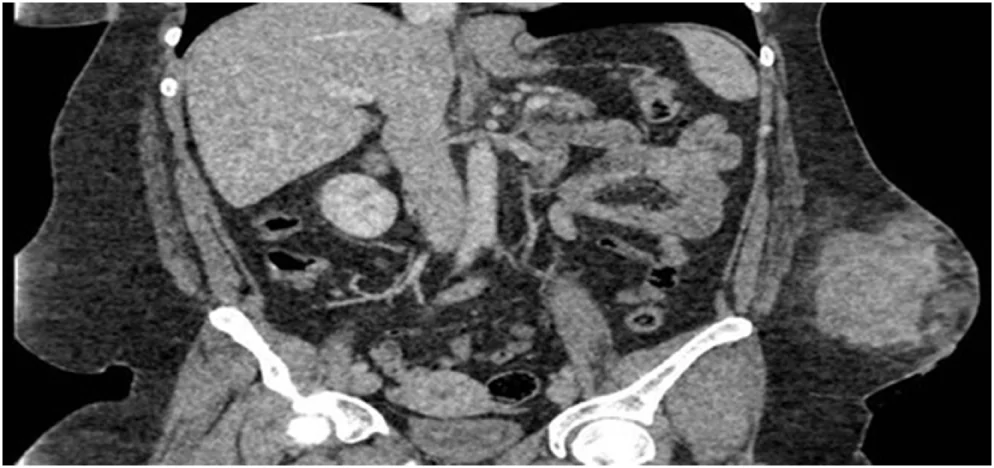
Contrast-enhanced CT scan of the abdomen on admission, showing a 4.7 × 10.8 × 8.9 cm hematoma.
